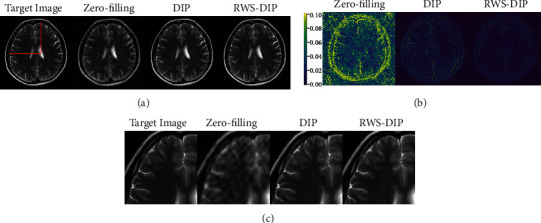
Comparison of reconstructions of the target MR image in Brain B using the radial undersampled mask with 20% sampling rate: (a) the target image and reconstruction results, (b) the corresponding error images, and (c) the corresponding zoom-in images.
